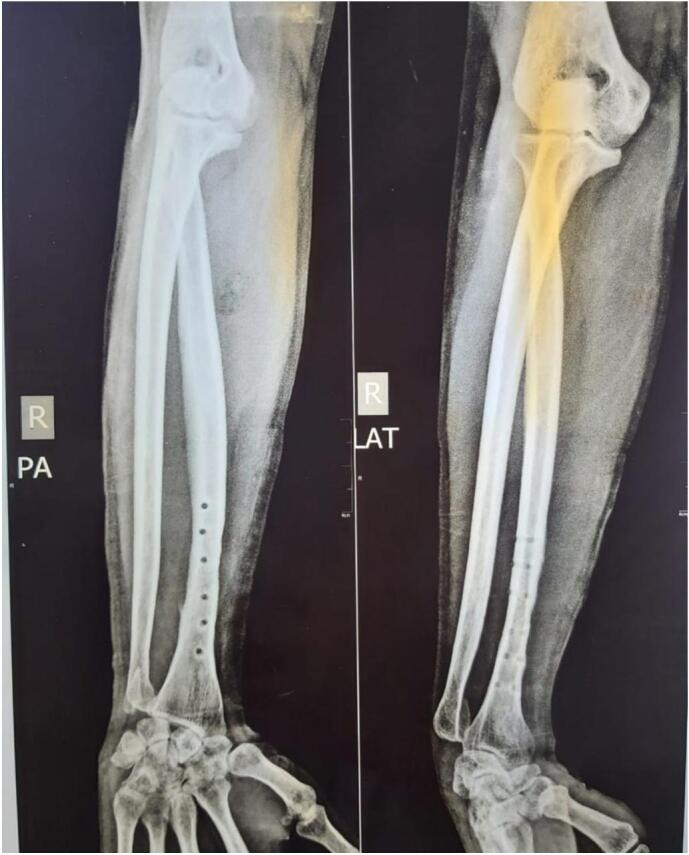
Preoperative MRI of the right wrist in coronal (a), axial (b), and sagittal (c) planes showing a dorsal dislocation of the right distal radioulnar joint and a triangular fibrocartilage complex tear.
